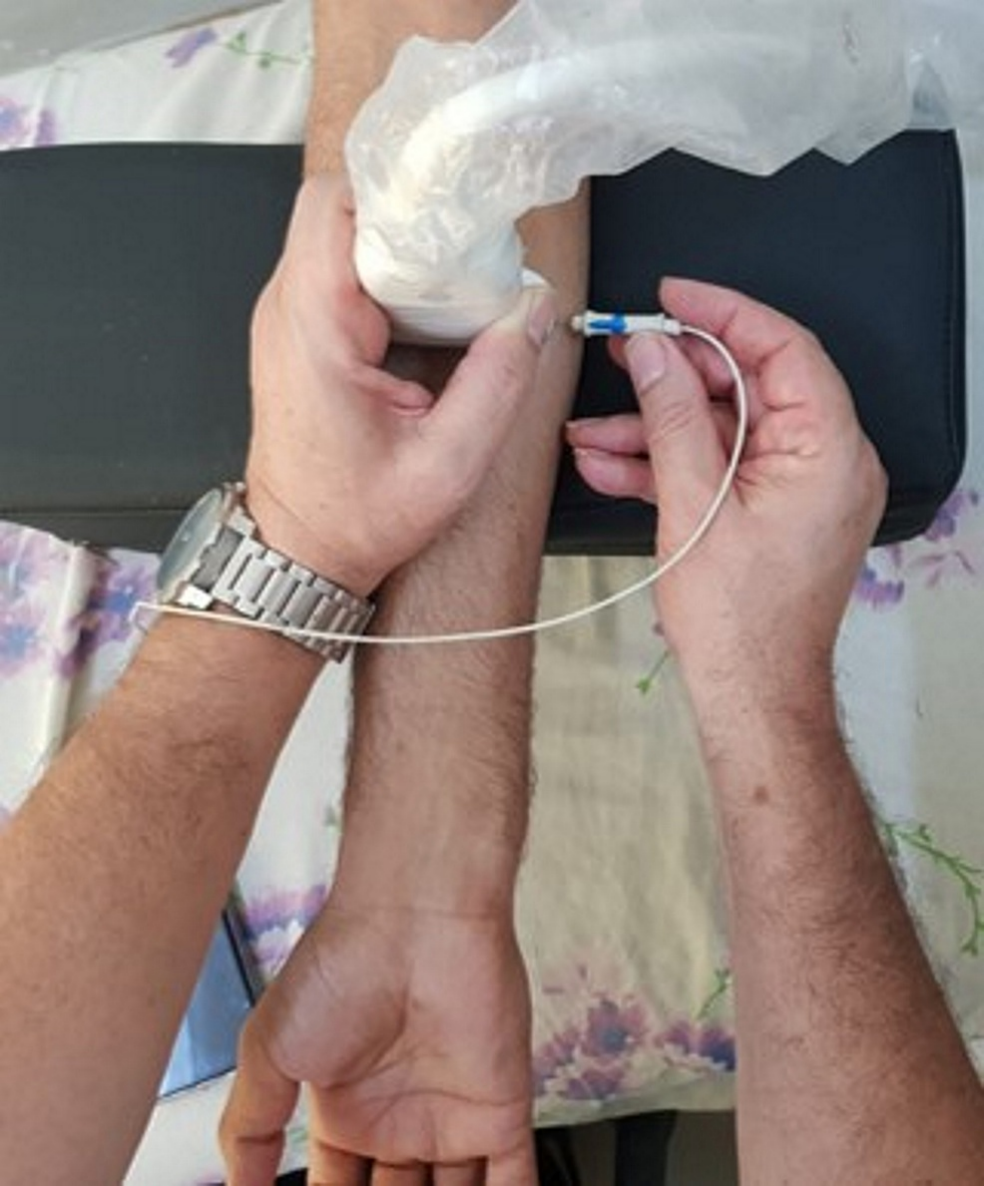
EMG needle insertion under US control. The US probe is placed at the junction of the upper and middle thirds of the anterior side of the forearm. The EMG needle is inserted at the medial edge of the forearm and 2 cm away from the US probe. This needle should then follow a sloped path to the FCRM in the plane of the US section so as to be entirely viewed: this is called the US in-plane technique. US: Ultrasound; FCRM: Flexor carpi radialis muscle; EMG: Electromyography. Note: In this figure, a medical resident has voluntarily given his consent for demonstration purposes.
